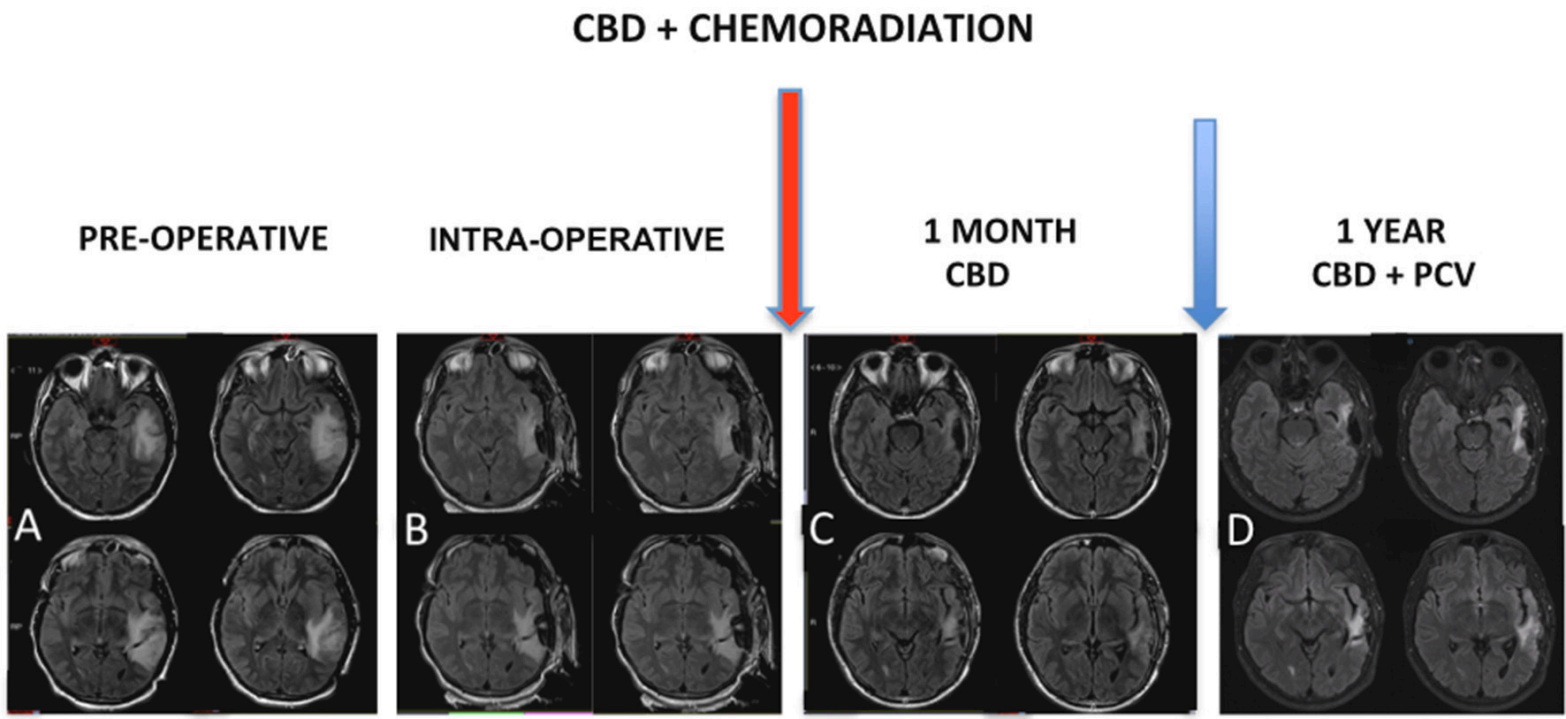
(A) MRI showed expansive infiltrative lesion predominantly subcortical of poorly defined contours, located in the left temporal lobe, involving the upper, middle and lower temporal gyrus, with increase of the left temporal gyrus cortex. The lesion compromises a large part of the temporal lobe and extends to the temporal isthmus, posterior aspect of the insula, and deeply to the trigeminal effigy of the left lateral ventricle. There is diffuse erasure of the regional cortical sulci and the Sylvian fissure, as well as slight compression over the atrium of the left lateral ventricle. (B) Intraoperative MRI. Red arrow: chemoradiation associated with cannabidiol in a dosage ranging from 100 to 200 mg/day. (C) MRI control right after chemoradiation was characterized post-operative changes associated with a significant reduction of the infiltrative components of the tumor. (D) Magnetic resonance control after 1 year characterized no evidence of disease progression.
